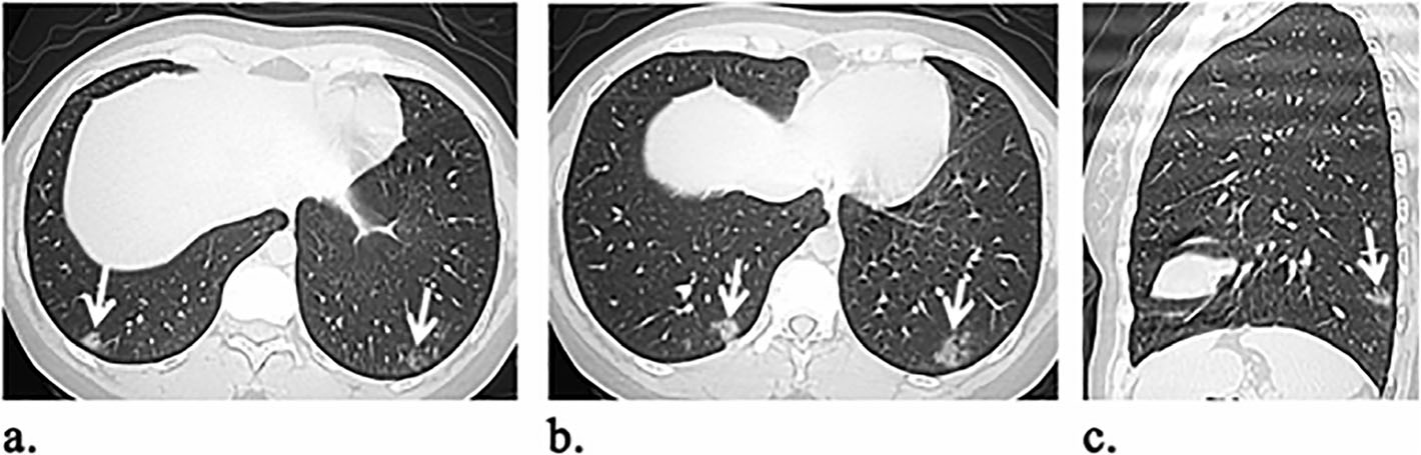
A 28-year-old female patient presented with significant myalgia and headache for 7 days. O_2_ saturation was 97%. a, b Axial and c sagittal unenhanced HRCT images reveal bilateral multifocal lower lobe lung infiltrations with small rounded patches of GGO and consolidation close to the pleura yet sparing it (white arrows)
